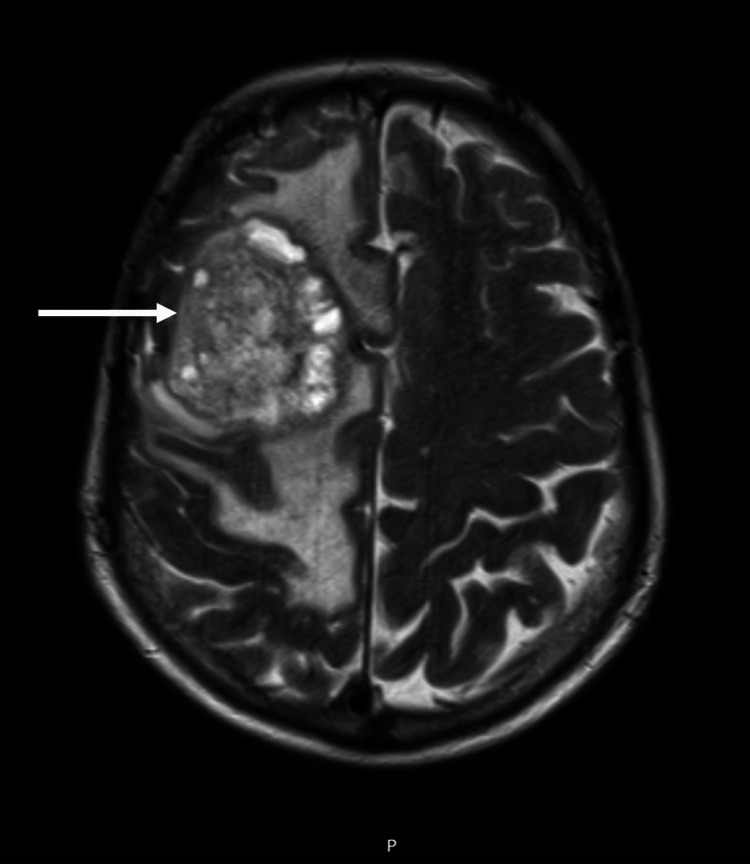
MRI showing a right frontal lesion of 4 cm in the right frontal lobe.
